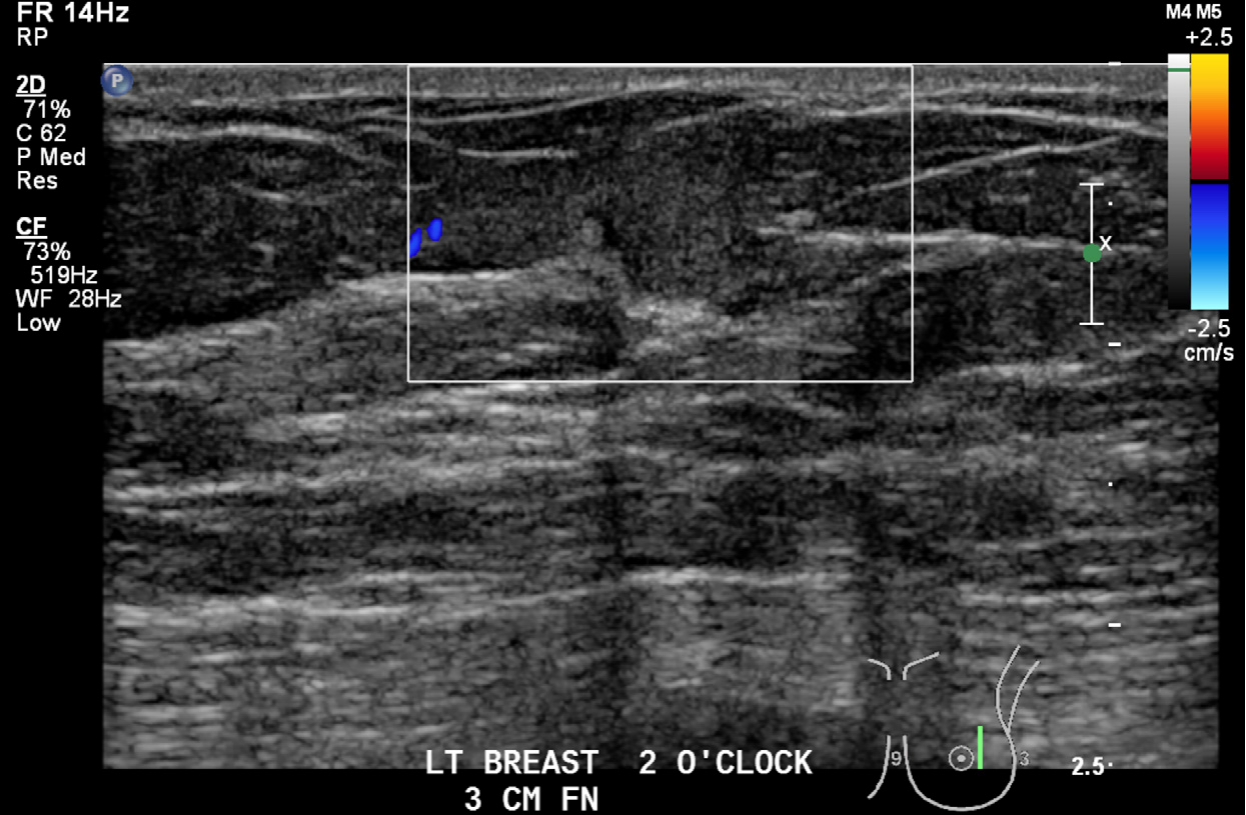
Lesion two with Doppler interrogation demonstrating avascular nature. Image courtesy of BreastScreen ACT.
